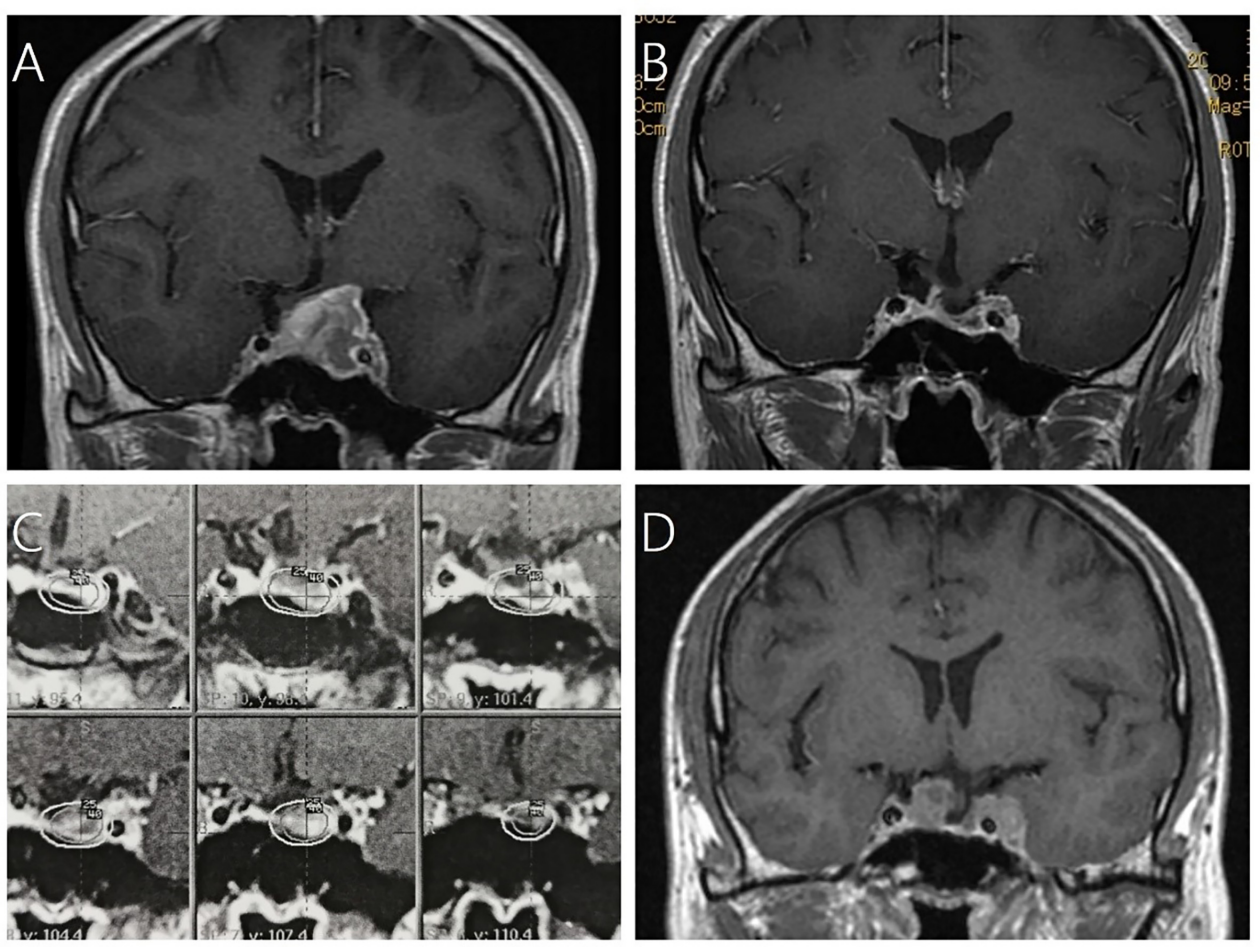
A 43-year-old male patient with residual NFPA after surgical resection received GKRS and developed tumor regrowth out of field at 71 months after GKRS. (A) contrast-enhanced coronal T1-weighted magnetic resonance imaging (MRI) scans showed pituitary adenoma. (B) MRI showed subtotal resection for pituitary adenoma after 3.5 months. (C) Dose distribution of adjuvant GKRS after surgical resection. (D) MRI showed tumor regrowth was either in the sellar as well as in the cavernous sinus out of field.
